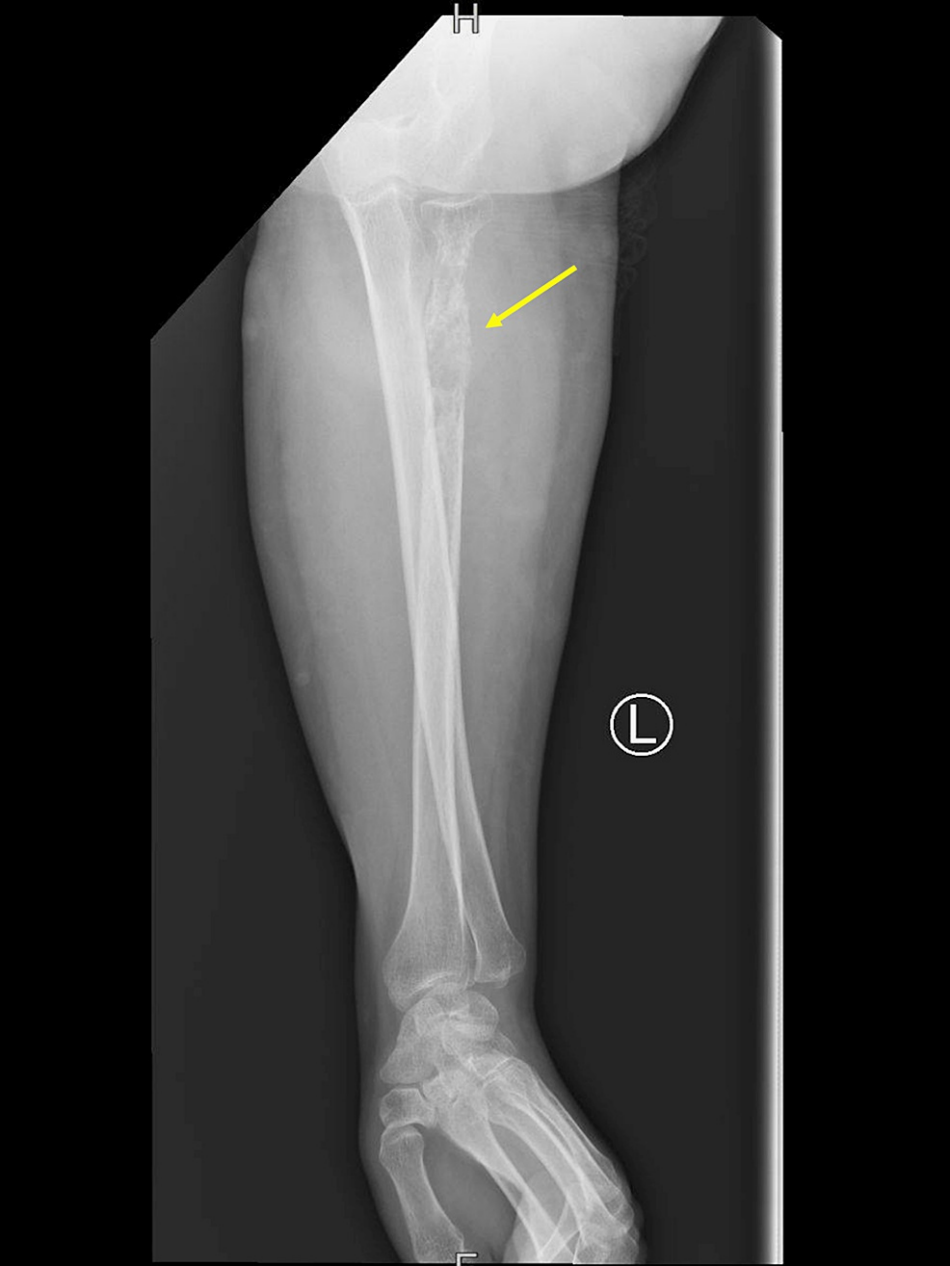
Anteroposterior (AP) X-ray view of the left forearm showing an erosive bony lesion
